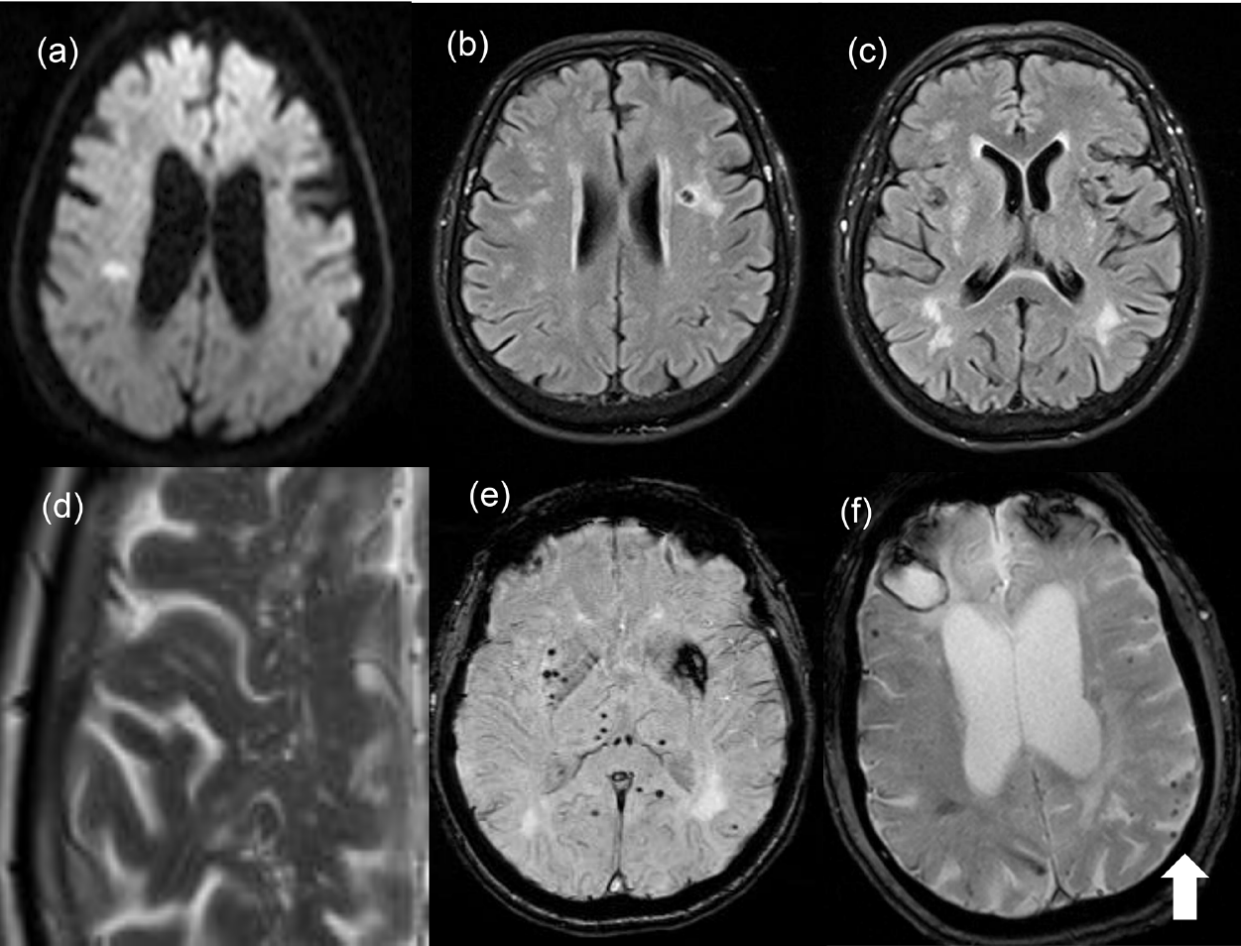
MRI appearances of SVD: Note the right side of the brain is represented on the left-hand side of the images: (A) An right hemisphere acute lacunar infarct visible as high signal on diffusion-weighted imaging; (B) a chronic lacunar infarct on the left visible as an area of low signal on FLAIR MRI; (C) white matter hyperintensities particularly in the posterior periventricular white matter; (D) a close-up of a T2-weighted MRI showing enlarged PVSs; (E) gradient echo MRI from a patient with sporadic hypertensive SVD showing multiple CMB visible as small dots of low intensity. These as predominantly subcortical, in contrast to mainly cortical CMB seen in CAA. There is also a larger area of low density in the left hemisphere representing changes from an old subcortical intracerebral hemorrhage; (F) gradient echo MRI from a patient with CAA showing multiple cortical CMB (arrowed), as well as a right frontal lobar intracerebral hemorrhage. © Hugh Markus.
